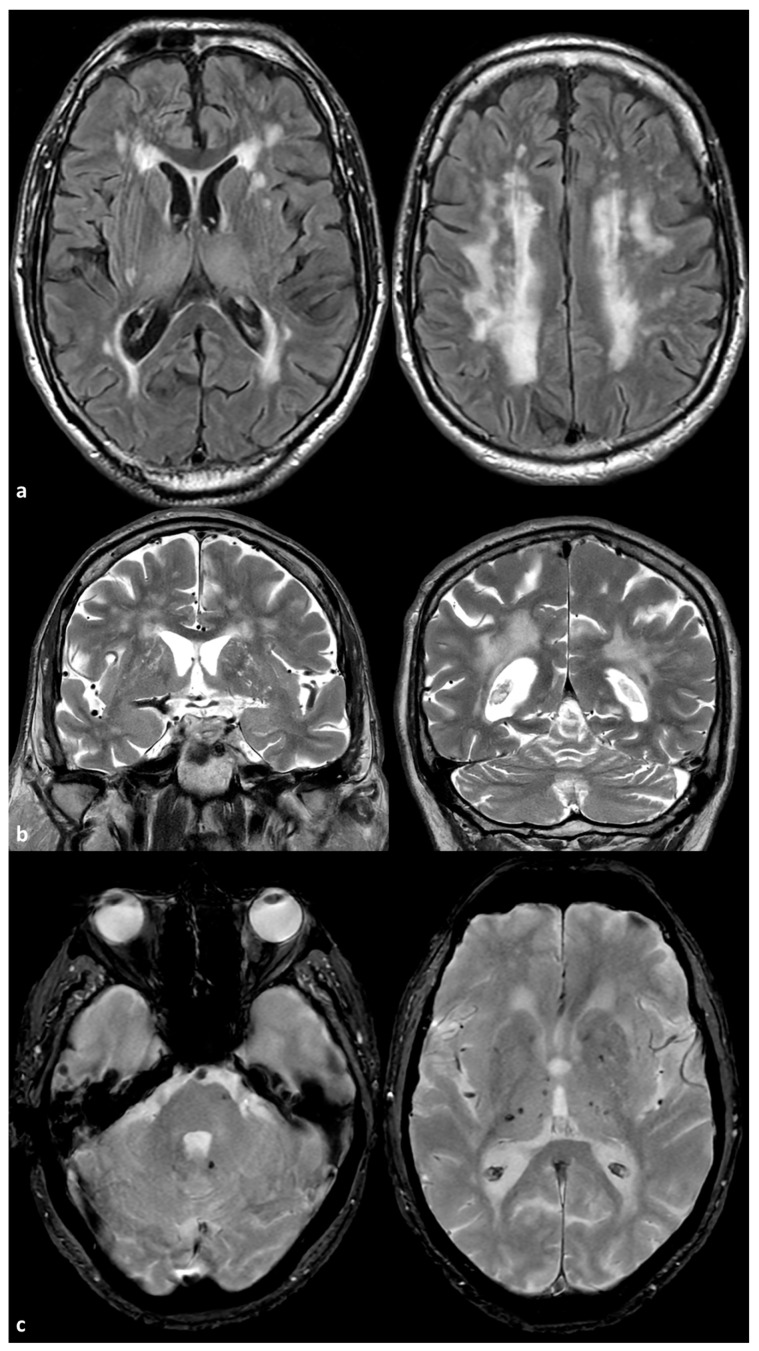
Brain Magnetic Resonance Imaging (MRI) of a 72-year-old man with a mutation ABCC6(NM_001351800.1):c.3840delG:(p.Lys1280AsnfsTer9) in heterozygosis. The patient has a very mild arterial hypertension and was sent to the neurological attention because of the occurrence of transient neurological deficit. In panel (a), some examples of axial Fluid Attenuated Inversion Recovery (FLAIR) images are provided at basal ganglia level (left image) and at centrum semiovale level (right image), showing symmetric, extensive, confluent white matter hyperintensities that are prevalent in the centrum semiovale. In panel (b), coronal T2W images show the enlarged perivasculae spaces in the basal ganglia on both sides. In panel (c), Gradient Recalled Echo (GRE) images show isolated pontine and deep, supratentorial, hypointense rounded signals similar to those observed for microbleeds.
